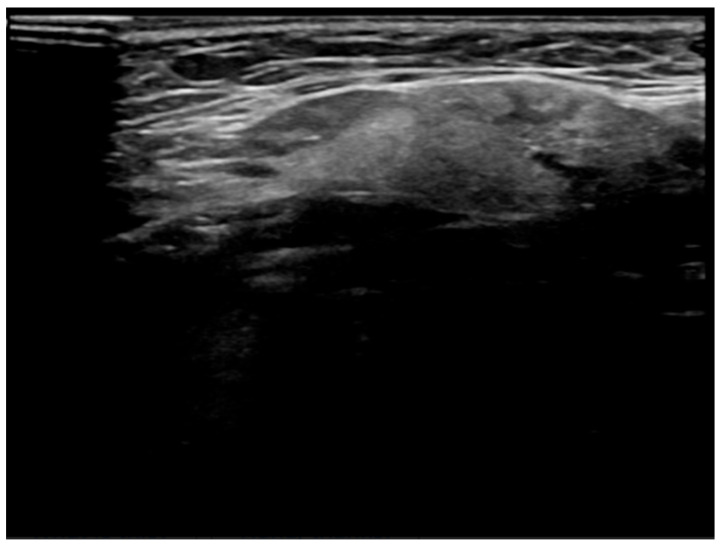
Patient Z—Follow-up ultrasound scan of affected SMG. The previously identified abnormal area in the left SMG shows significant spontaneous resolution on follow-up imaging.
